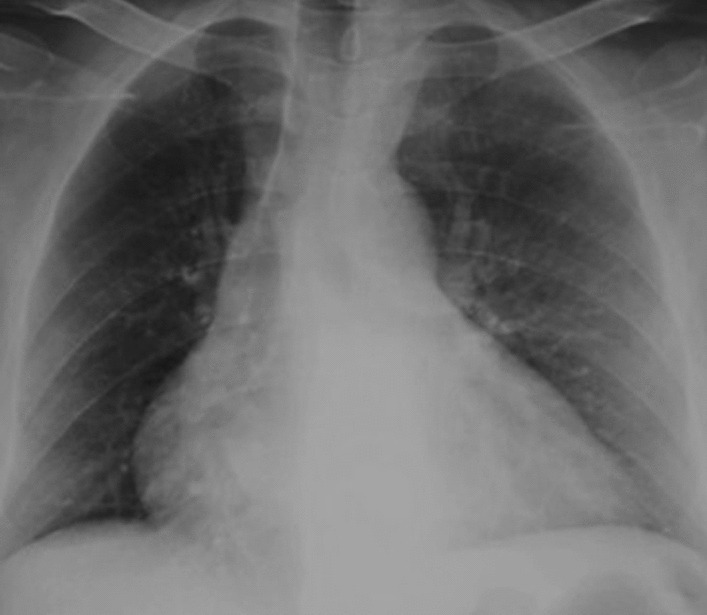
Chest X-ray showing signs of left atrial enlargement
